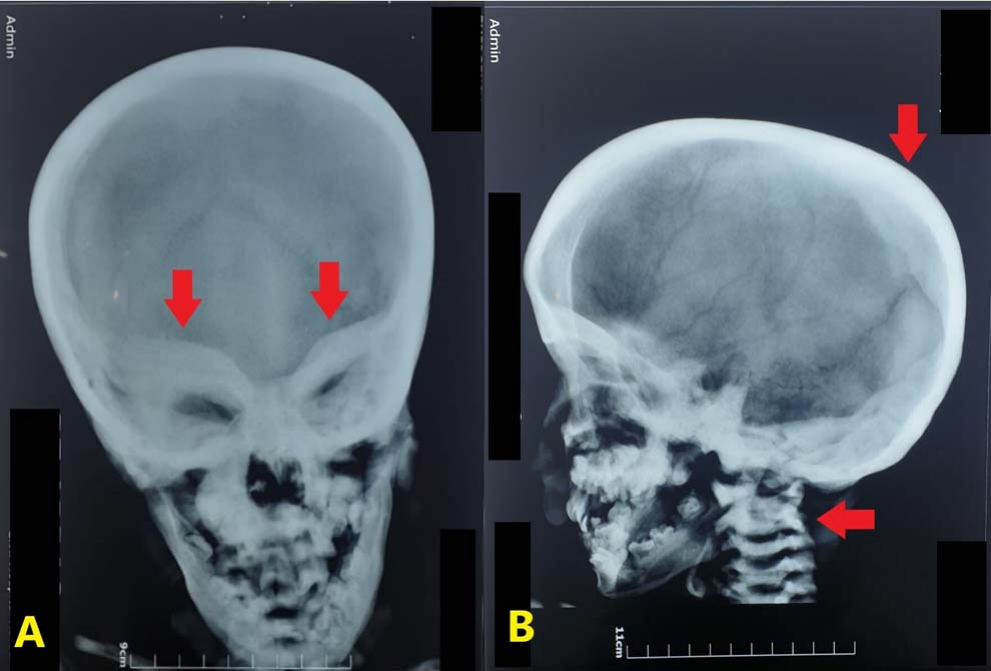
Skull radiographs show (A) periorbital sclerosis (arrows) and (B) thickening of the calvarium and the visualized cervical vertebrae (arrows).
